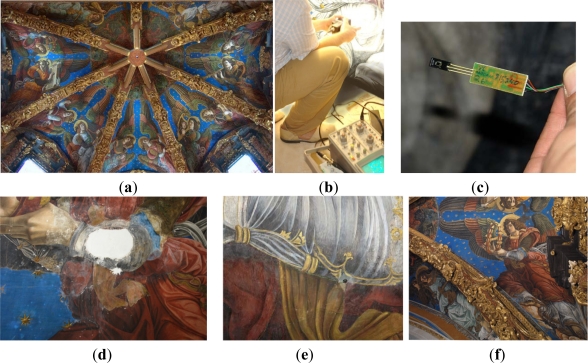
(a) Overview of the vault of the Valencia Cathedral; (b) Manual scan looking for the sensors insertion points in frescoes; (c) Humidity sensor detail; (d) Forearm detail of (f) before restoration; (e) In the center of the picture, a sensor is inserted by the ultrasound technique in (d); (f) Detail of the vault after the restoration.
